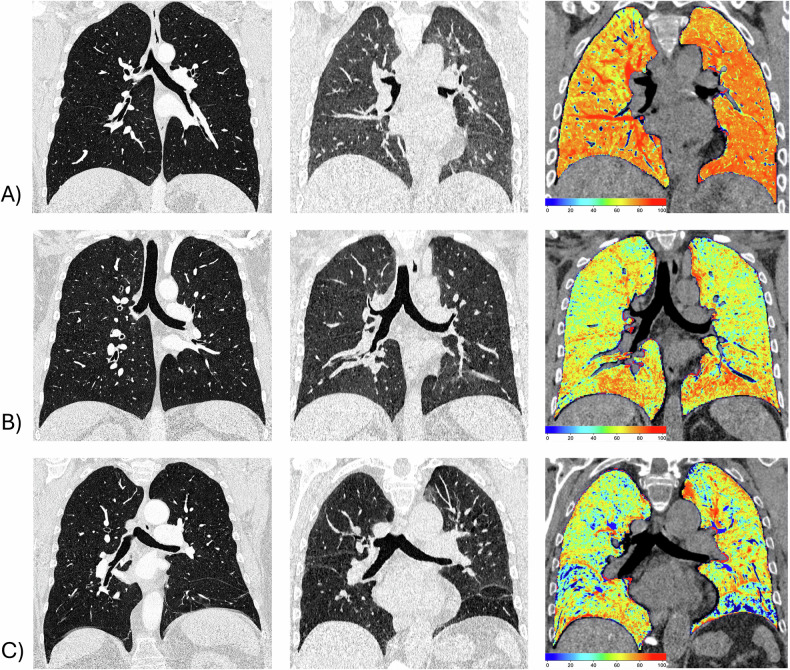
Coronal images obtained at inspiration (left), expiration (middle), and CT-derived ventilation maps (right) from three participants of different ages, demonstrating decreasing ventilation and increasing heterogeneity (increasing SD) with increasing age. A A 33-year-old participant demonstrating high and homogeneous ventilation of 76.6% ± 26.4%. B A 45-year-old participant showing moderate ventilation of 51.9% ± 23.2%. C A 76-year-old participant exhibiting reduced and heterogeneous ventilation of 40.7% ± 38.7%
